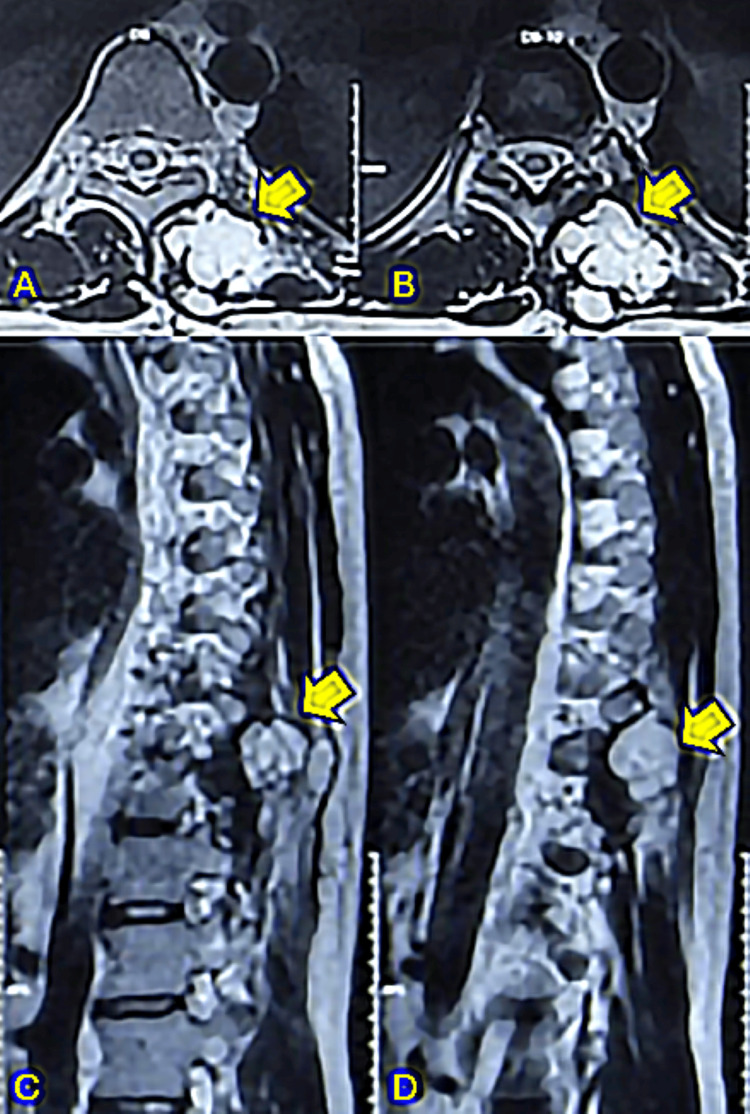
T2-weighted MRI showing the chondrosarcoma at levels T9, T10, and T11 6A and 6B: T2-weighted MRI axial cuts showing a well-defined, lobulated, hyperintense lesion predominantly involving the left paraspinal muscles at the T9, T10 vertebral body level with associated involvement of the T9, T10, T11 spinous processes, left transverse processes and pedicles of the T9, T10 vertebrae, and posteromedial aspects of the respective left ribs. 6C and 6D: MRI T2-weighted sagittal cuts showing a well-defined, lobulated, hyperintense lesion involving the posterior elements and paraspinal muscle at T9, T10, and T11.
